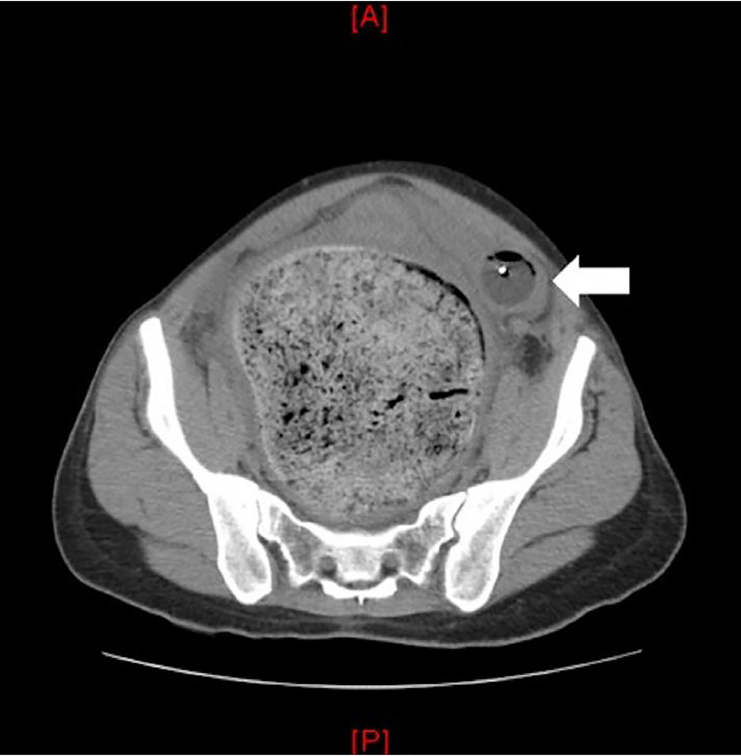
Computed tomography of the abdomen in the axial plane demonstrating stool burden causing significant displacement of the bladder as noted by the urinary catheter (arrow).
